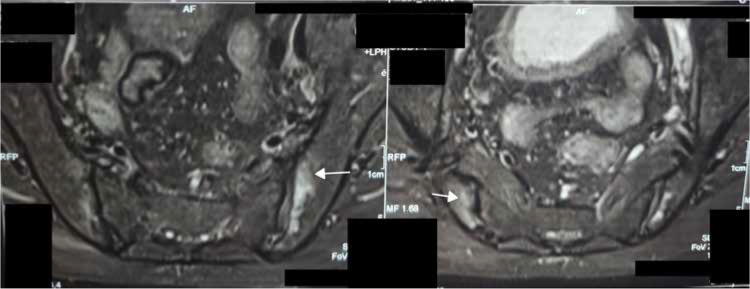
MRI of the sacroiliac joints in STIR sequence showing bilateral sacroiliitis characterised by increased signal intensity depicting bone marrow oedema in bilateral sacroiliac joints.
